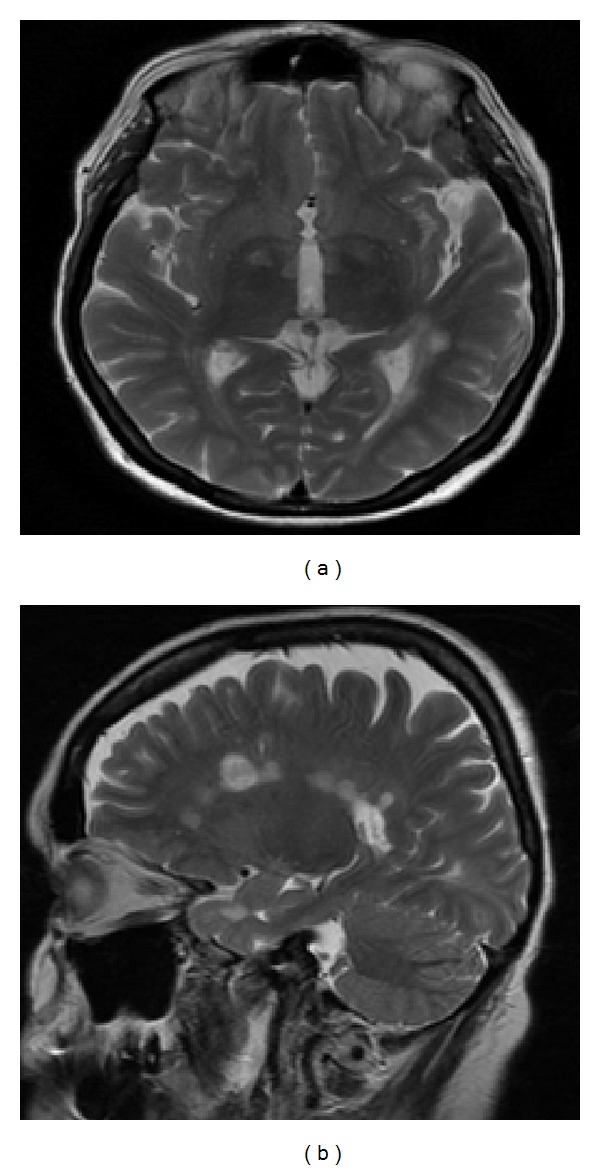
MR of the head; T2-weighted images (axial view (a) and sagittal view (b)) demonstrate numerous plaques in white matter distribution.
